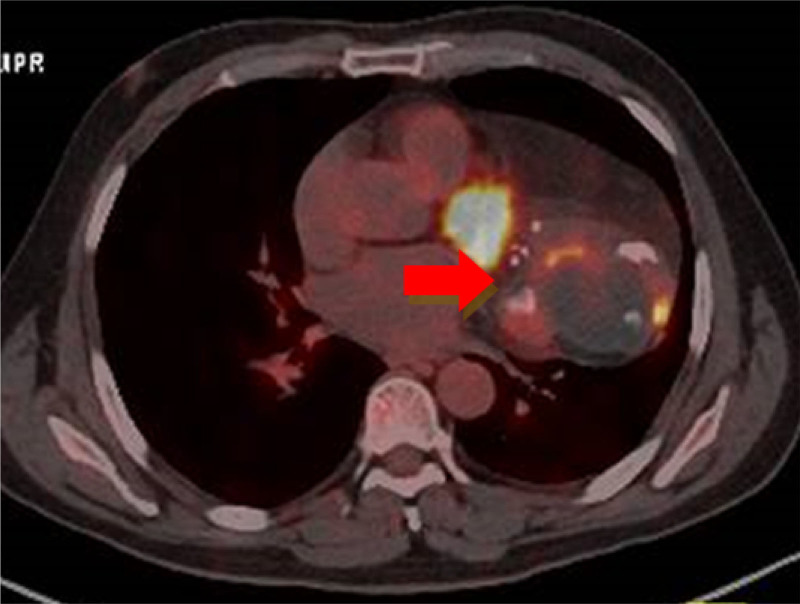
PET. PET revealed FDG accumulation in the tumor. FDG = Fluorodeoxyglucose, PET = positron emission tomography.
